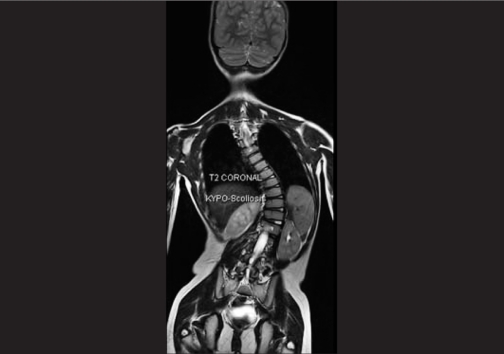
MRI spine of case 1, T2 coronal
